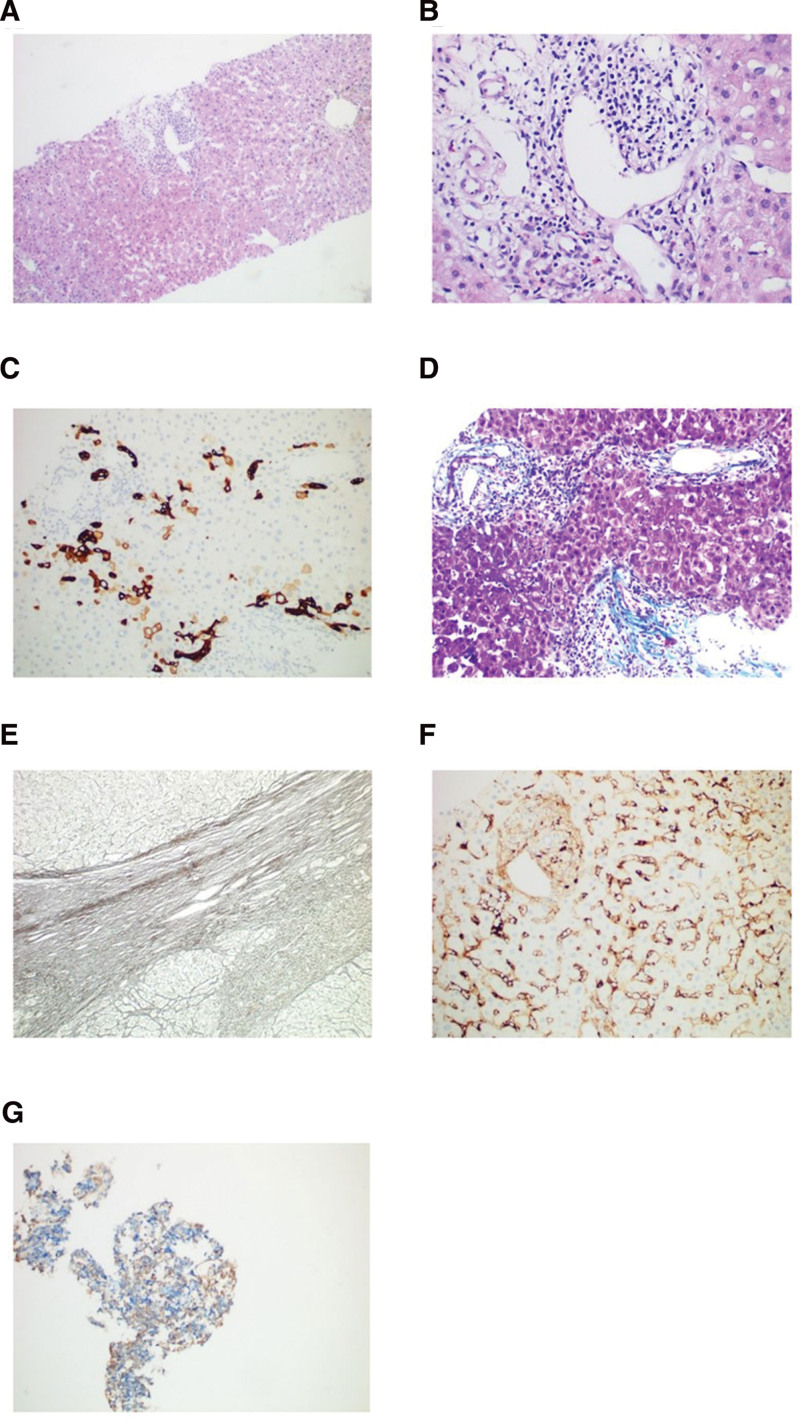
Upper abdomen MRI + MRCP in October 2023. (A) MRI: The uncinate process of the pancreas is still enlarged, but the volume has decreased compared to September 2021 (Fig. 1F). (B) Comparing to the situation of intrahepatic and extrahepatic common bile ducts in September 2021 (Fig. 1B), the narrowing of the hepatic portal bile duct has worsened. (C) The capsular sign of the pancreas is not obvious, and the pancreatic volume has decreased compared to September 2021. (D) The extent of common bile duct dilation has reduced compared to September 2021.
